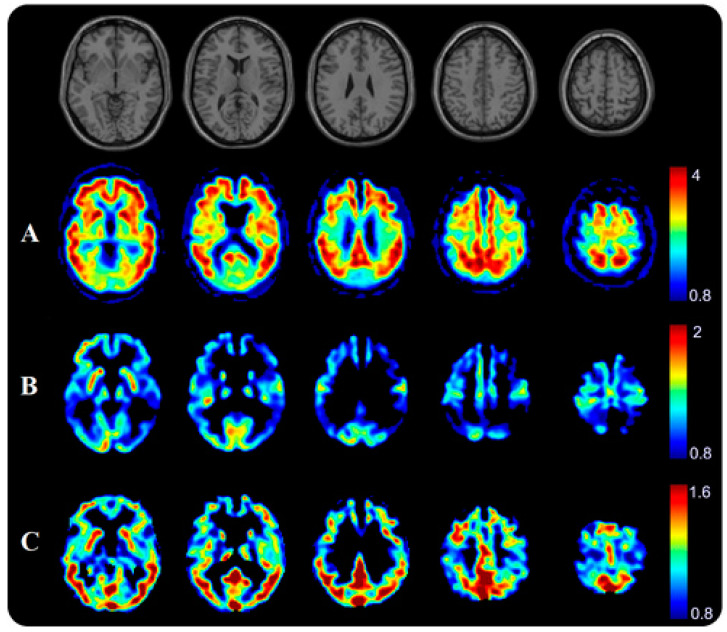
Serial transverse brain sections from (A) [11C]PiB-PET, (B) [18F]FDG-PET, and (C) [11C]PBB3-PET for a patient with Alzheimer’s disease (female, 61 y, MMSE = 14). The color bar shows standardized uptake value ratios (SUV-Rs) with the cerebellar cortex as reference. The single-subject T1-weighted structural template supplied with SPM12 is also presented to provide a detailed view of the brain structures. Department of Nuclear Medicine. 2023. “Axial Slices C11-PiB, F18-FDG, C11-PBB3”. Department of Nuclear Medicine, University Hospital Ulm.
